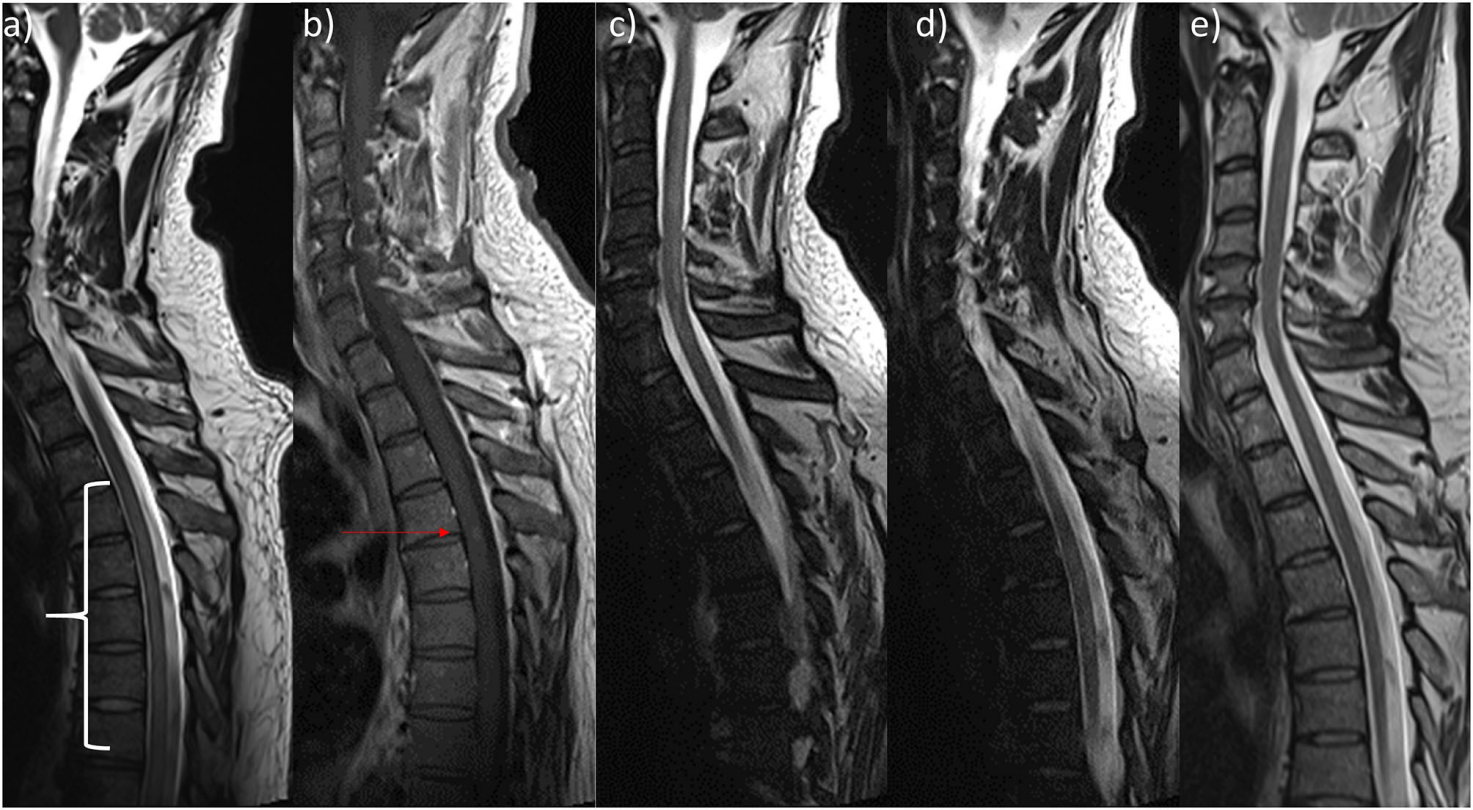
Sagittal Magnetic resonance imaging of patient's spine. (a) On admission there was discrete signal enhancement spanning from T4 to T11 in T2-weighted sequences. (b) 2 weeks later, there was a discrete contrast enhanced signal at the height of T4/5 in T1-weighted sequences. (c,d) Prior to discharge, T2-weighted sequences showed (c) a new cervical lesion spanning from C3 to C7 and the unchanged signal enhanced thoracic lesion (d). (e) Resolvance of myelopathic changes 1 year after initial admission.
